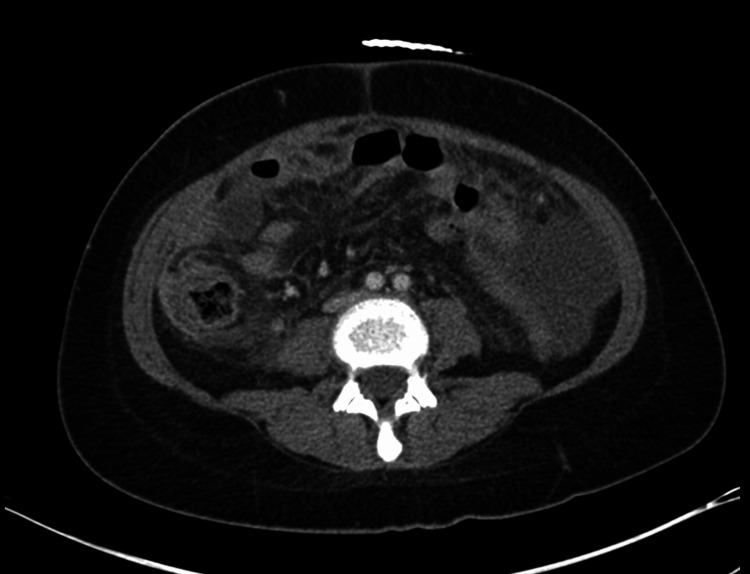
Axial images from CT abdomen pelvis with diffuse peritoneal thickening/enhancement consistent with peritonitis, prominent in R peritoneal reflection CT: computed tomography
